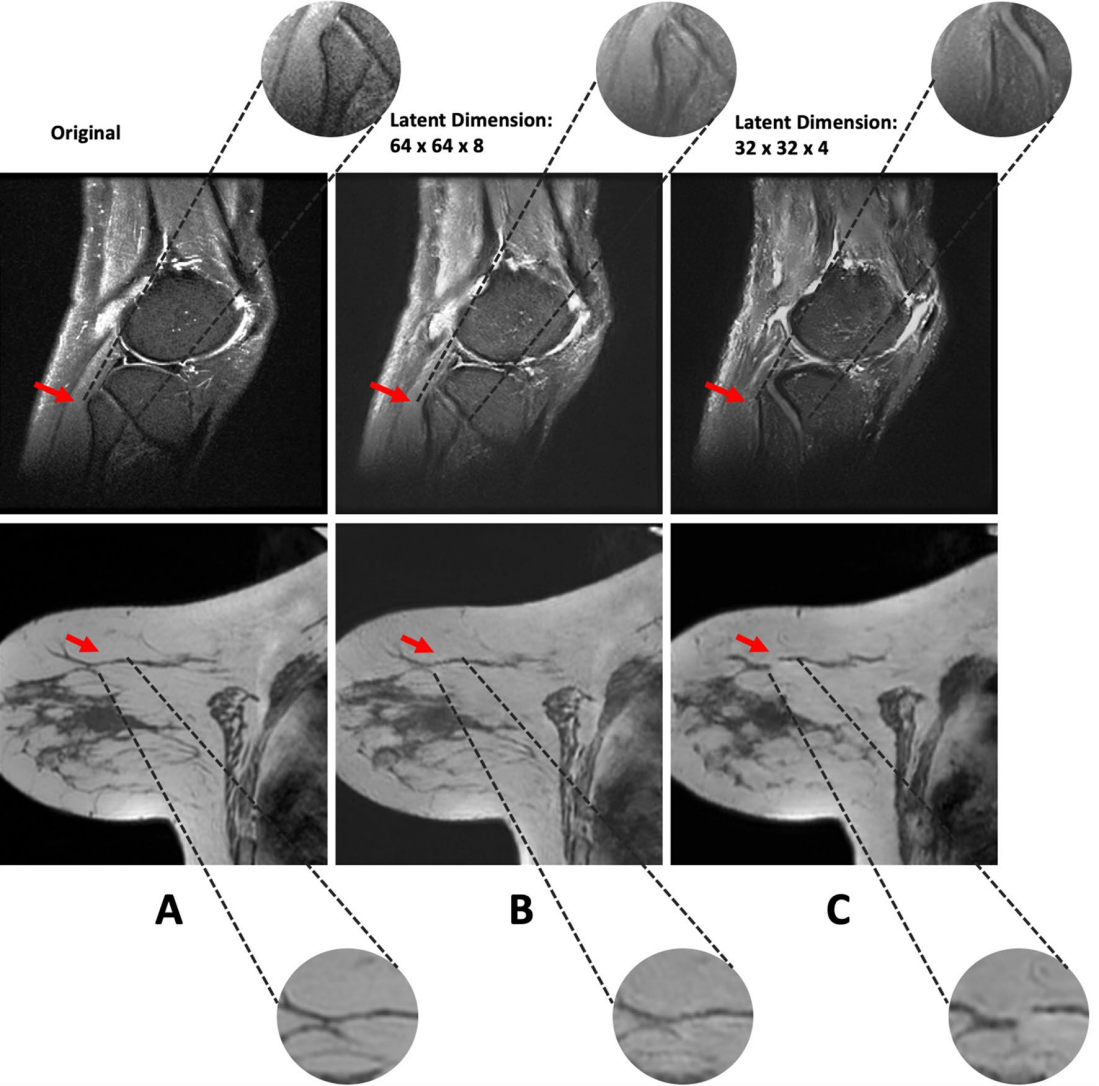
Comparison of the reconstruction quality of the VQ-GAN autoencoder when using different compression factors for two different samples. A latent dimension of 64 × 64 × 8 (i.e., a compression factor of 4 in each dimension, (B)) allows for a detailed reconstruction of the original image (A) by largely upholding anatomic consistency. A compression factor of 8 (i.e., a latent dimension of 32 × 32 × 4, (C)) distorts the fibular bone structure in knee MRI studies (upper row of images) and the fibroglandular tissue structure in breast MRI studies (lower row image). Adjacent, please find zoomed close-up views of the anatomic region of interest.
